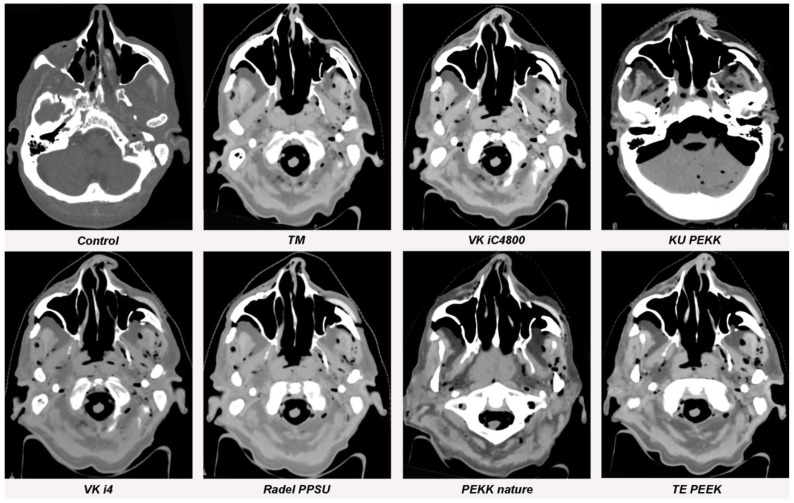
Streak artifacts of TM, VK iC4800, KU PEKK, VK i4, Radel PPSU, PEKK nature and TE PEEK in CT images in axial orientation versus control. Artifacts of each implant were measured at three different anatomical landmarks: (1) the zygomatic soft tissue, (2) the temporal muscle and (3) the parotid gland.
